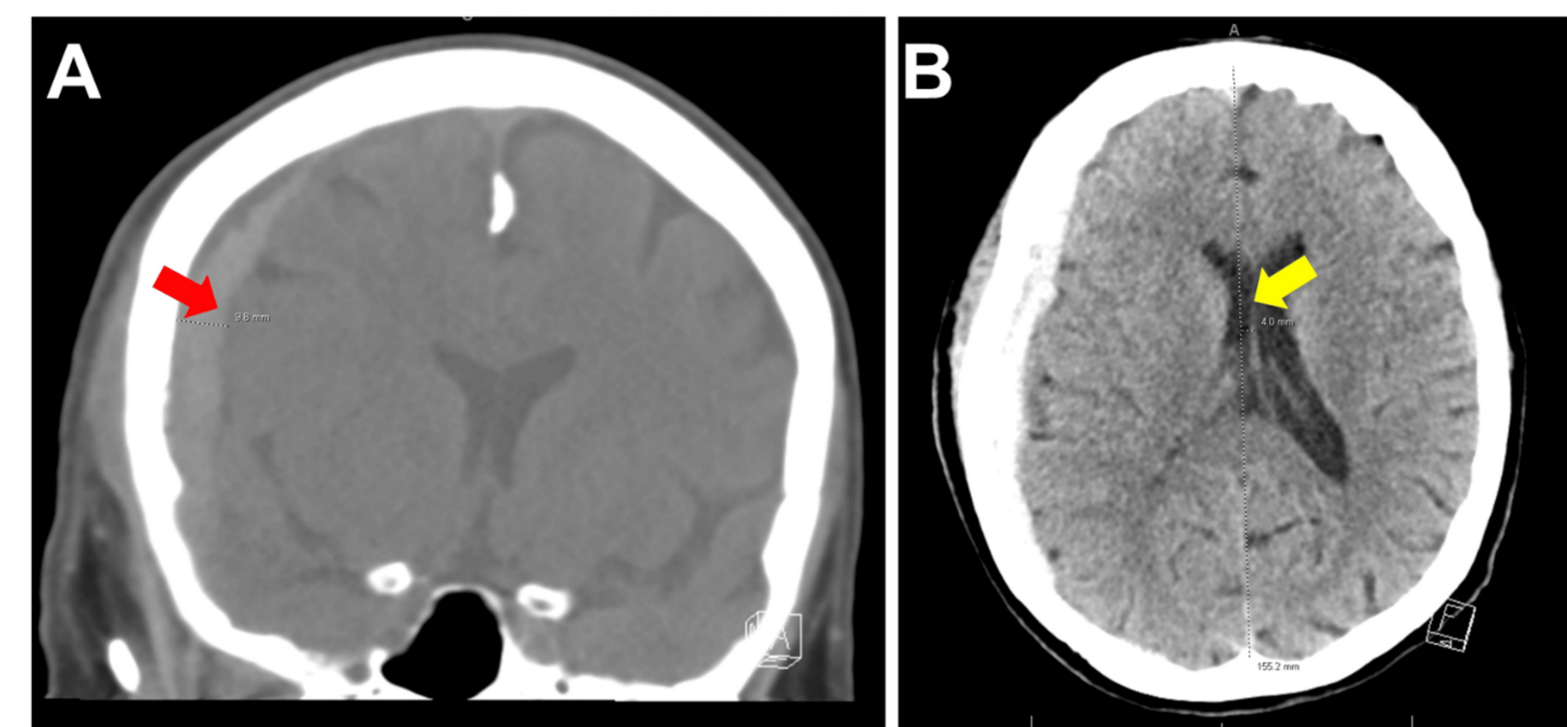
(A) Patient's computed tomography scan of the brain in coronal view demonstrates subdural hemorrhage without fracture or contusion of the skull (red arrow). (B) Axial view of computed tomography scan of the brain provides evidence of a 4-millimeter midline shift (yellow arrow).
